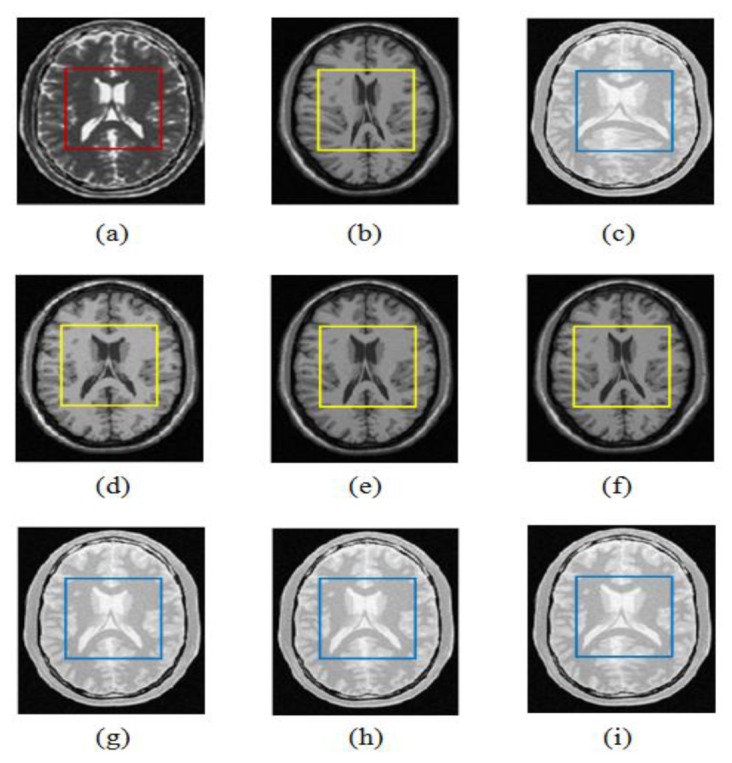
Non-rigid multi-modal registration results for the wldWSSIM-NMI, wldNSSD-NMI and ESSD-NMI methods operating on T1-T2 and PD-T2 weighted MR images. (a) T2 (the reference image); (b) T1 (the float image which is deformed by compressing mainly in the lateral ventricle marked with a yellow square.); (c) PD (the float image which is deformed by expanding mainly in the lateral ventricle marked with a blue square.); (d) wldWSSIM-NMI (T1-T2); (e) wldNSSD-NMI (T1-T2); (f) ESSD-NMI (T1-T2); (g) wldWSSIM-NMI (PD-T2); (h)wldNSSD-NMI (PD-T2); (i) ESSD-NMI (PD-T2).
